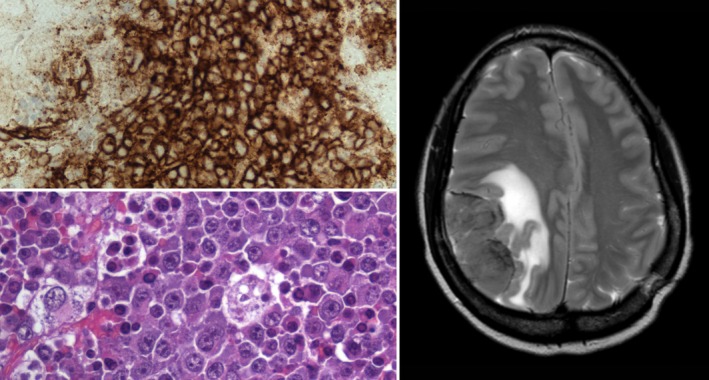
A 29‐year‐old woman presented with severe headaches, vomiting and drowsiness in January 2016. A computed tomography scan of the brain at the time showed bilateral subdural haematomas with midline shift, sub‐falcine herniation and hydrocephalus. The patient underwent a burr‐hole procedure with surgical evacuation of the haematomas. At the time of surgery, an extradural mass was noted and biopsied.
